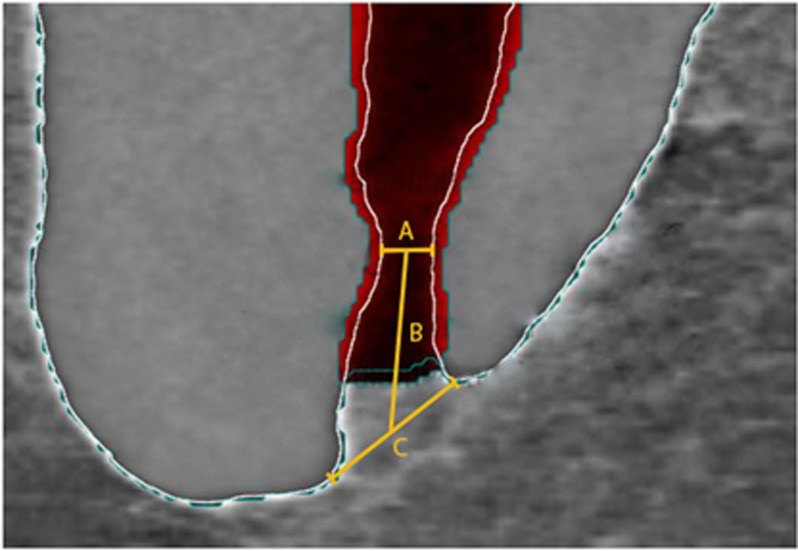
Micro-computed tomography representation of the distances measured at the apical foramen region. A, diameter (narrow) of the physiological foramen. B, distance between the physiological and anatomical foramen. C, diameter (wide) of the anatomical foramen.
